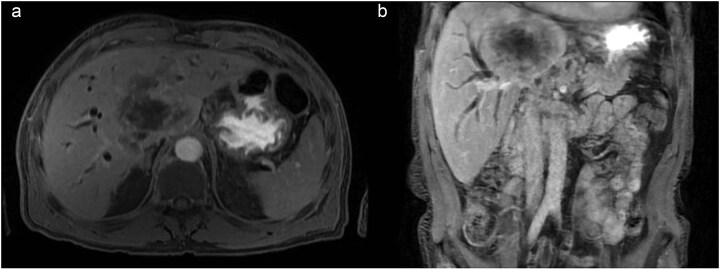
(a) Axial contrast enhanced CT scan shows a central infiltrative lesion in the hepatic parenchyma, affecting segments I, IV, and VIII, measuring 11.0 × 8.8 cm. (b) Coronal contrast enhanced CT scan the mass causing obstruction of the bile duct at the confluence of the hepatic ducts and involvement of segmental ducts of both lobes.
